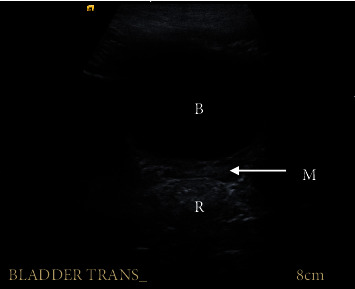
Müllerian remnant viewed on surveillance pelvic ultrasound scan. Key: M: Müllerian remnant; B: bladder; R: rectum.
